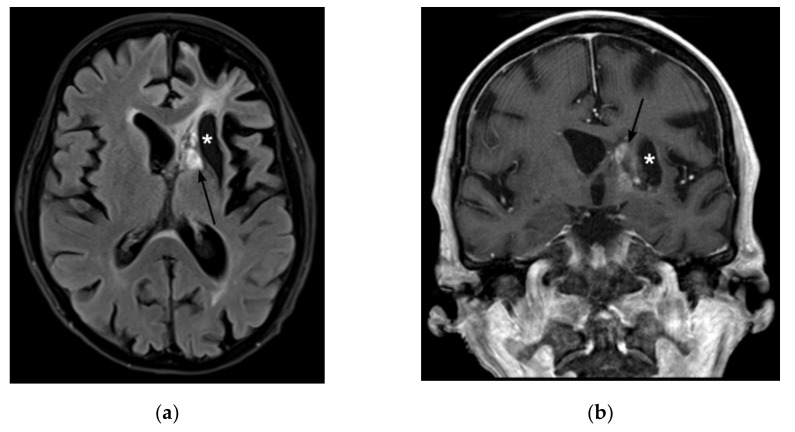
Magnetic Resonance Imaging (MRI) on day 251 after initial admission to the hospital (a) Fluid attenuated inversion recovery (FLAIR) shows regressing lesions with mixed T2 signal (arrow) and a hypointense tissue defect at the former site of the abscesses (star). (b) Contrast-enhanced T1-weighted image in corona shows regressive enhancement of the lesions.
